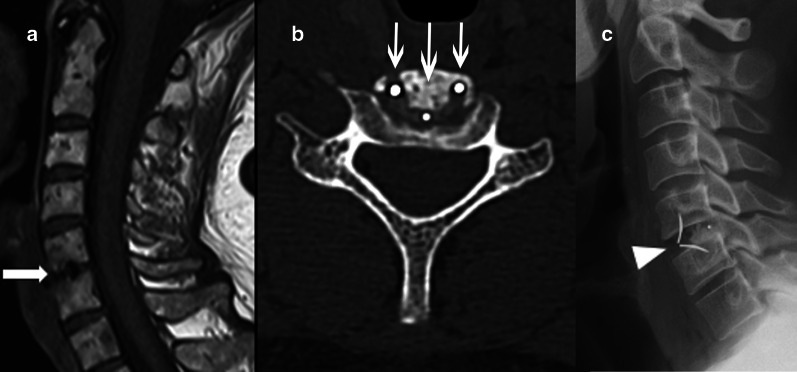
Anterior fusion procedure with interbody cage spacer due to trauma (a and b) and degenerative disc disease (c). Sagittal T1W MR image (a) demonstrates mild magnetic susceptibility artifact produced by the metallic cage (arrow) at the C5-6 level, making it difficult to evaluate. Axial CT image (b) demonstrates optimal placement of the interbody spacer in the center of the vertebral body, as delineated by radiopaque markers (thin arrows). A posterior marker located at least 2 mm anterior to the posterior edge of the adjacent vertebral body indicates the spacer is in a good position. Lateral radiograph (c) from another patient demonstrates a stand-alone cage with self-locking plates (arrowhead) attached to the vertebral bodies to provide stability. The spacers’ purpose is to promote fusion while maintaining spine alignment and support
